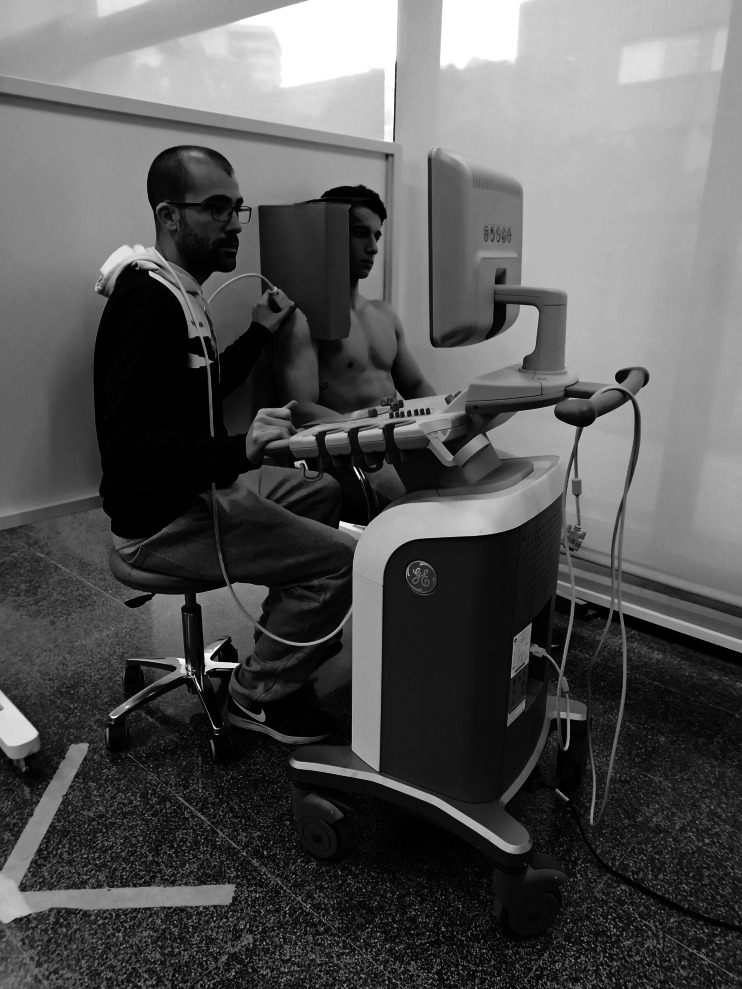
Ultrasound measurement. Photo credit: Rodrigo Martín-San Agustín.
